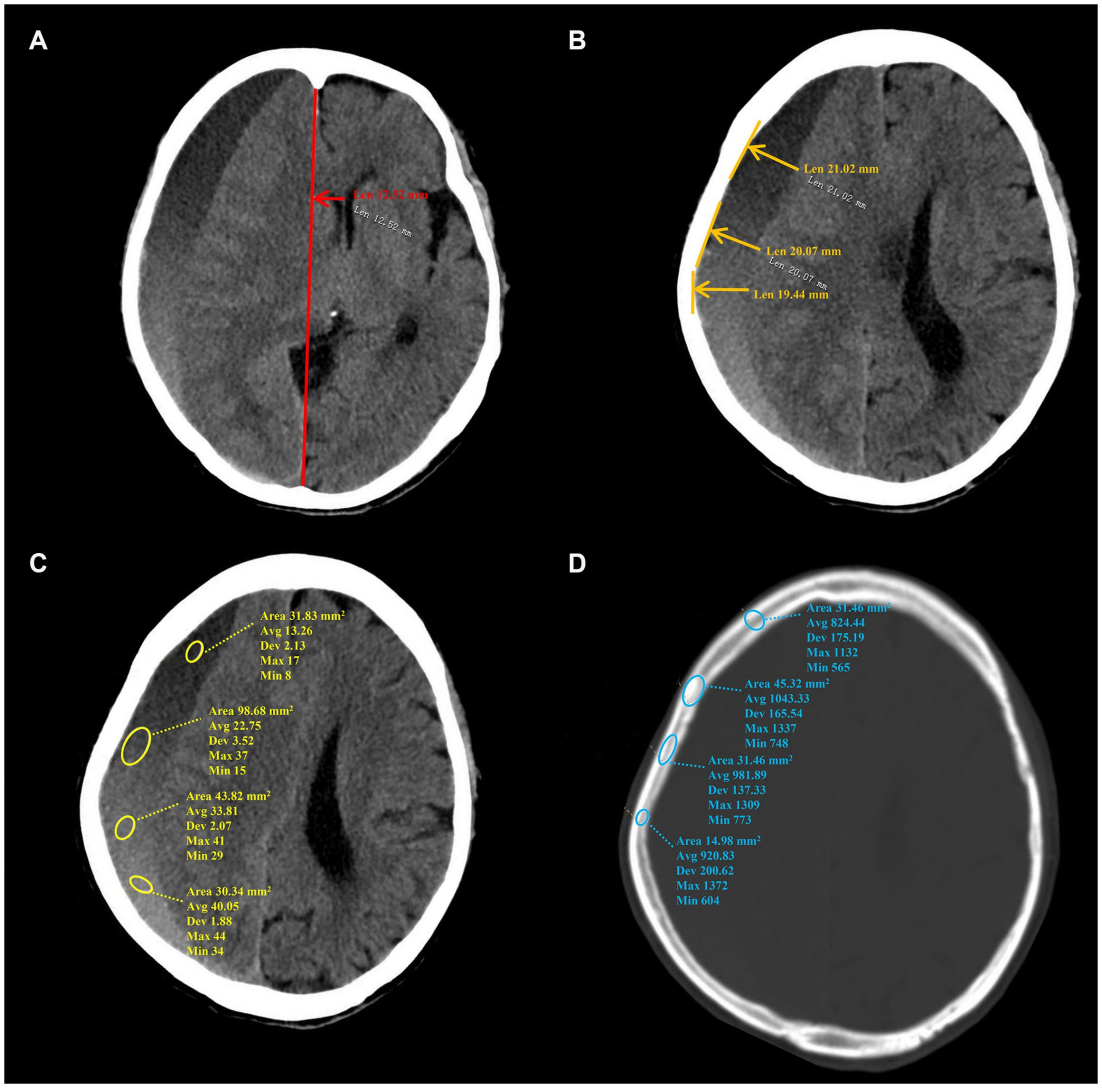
The data measurement method on CT. (A–D) Show the measurement method of MS, HT, hematoma density and skull density, separately. MS, midline shift; HT, maximal hematoma thickness.
